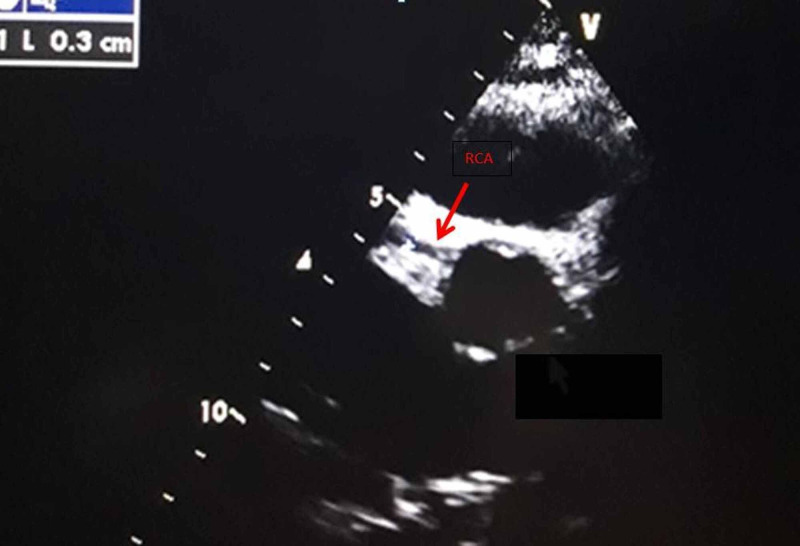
Echocardiography: parasternal short axis view showing right coronary artery (RCA) 3mm
